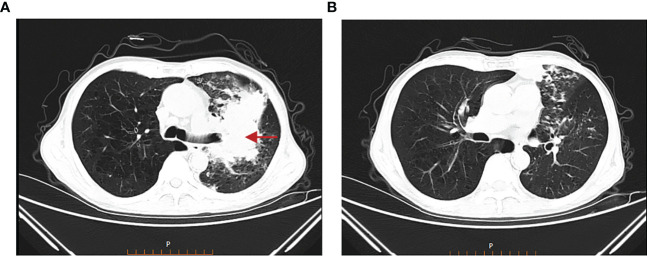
Chest CT on 05 March 2022. (A) Truncated bronchi in the upper lobe of the left lung, multiple small patchy and nodular high-density shadows in the surrounding lung. (B) Multiple small nodules and small patchy high-density shadows in the lower lobe of the left lung.
